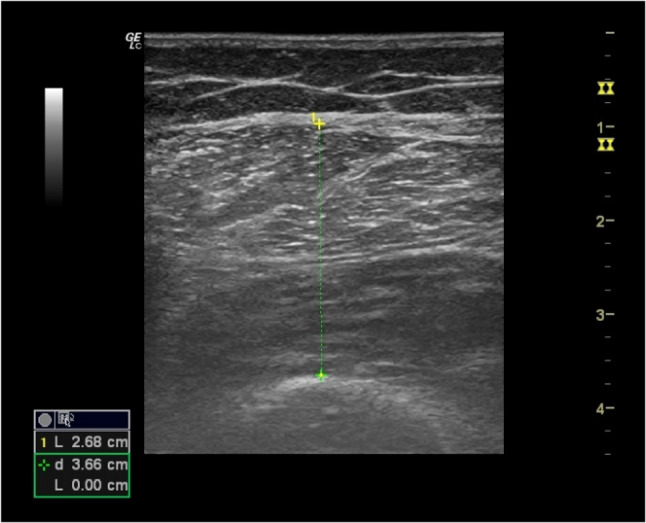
Ultrasound measurement of anterior thigh muscle thickness (ATMT). The measurement represents the combined thickness of the rectus femoris and vastus intermedius muscles at the midpoint between the anterior superior iliac spine and the upper border of the patella
